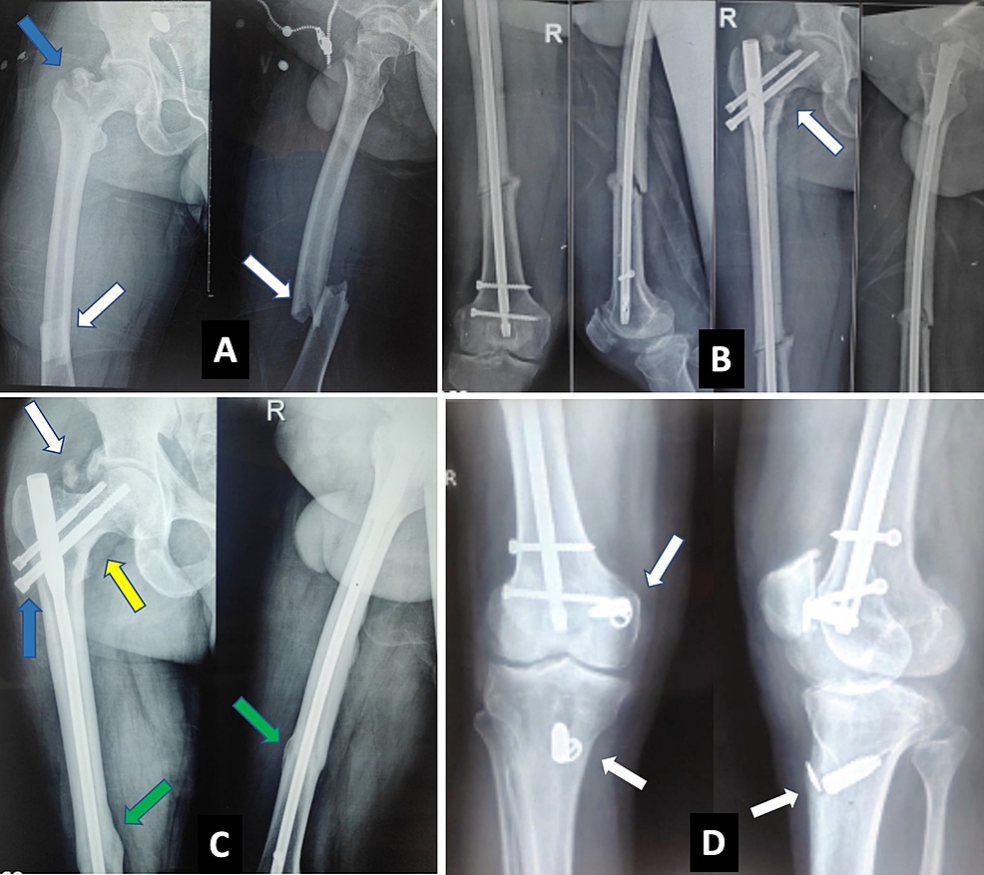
(A) Preoperative X-ray of a patient with intertrochanteric fracture (blue arrow) with femoral shaft fracture (white arrow) at the infra-isthmic level. (B) Immediate postoperative X-ray of the patient after cephalomedullary nailing showing fixation of intertrochanteric fracture in varus (white arrow). (C) Follow-up X-ray at 12 months showing union of the femoral shaft (green arrows). Heterotrophic ossification (white arrow), proximal screw backout (blue arrow), and varus malunion of the intertrochanteric fracture (yellow arrow) can also be seen. (D) The patient also had posterior cruciate ligament injury, for which he underwent reconstruction (white arrows). However, it failed later secondary to fresh trauma, leading to residual knee laxity.
